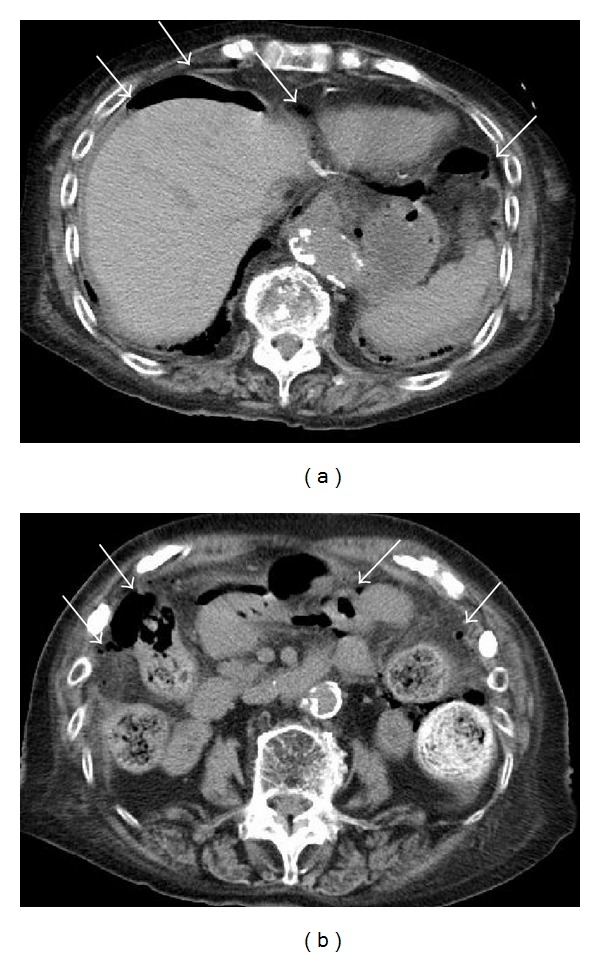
Abdominal computed tomography revealed the presence of ascites, free air in the abdominal cavity, and hard stools in the left-sided colon ((a), (b)).
